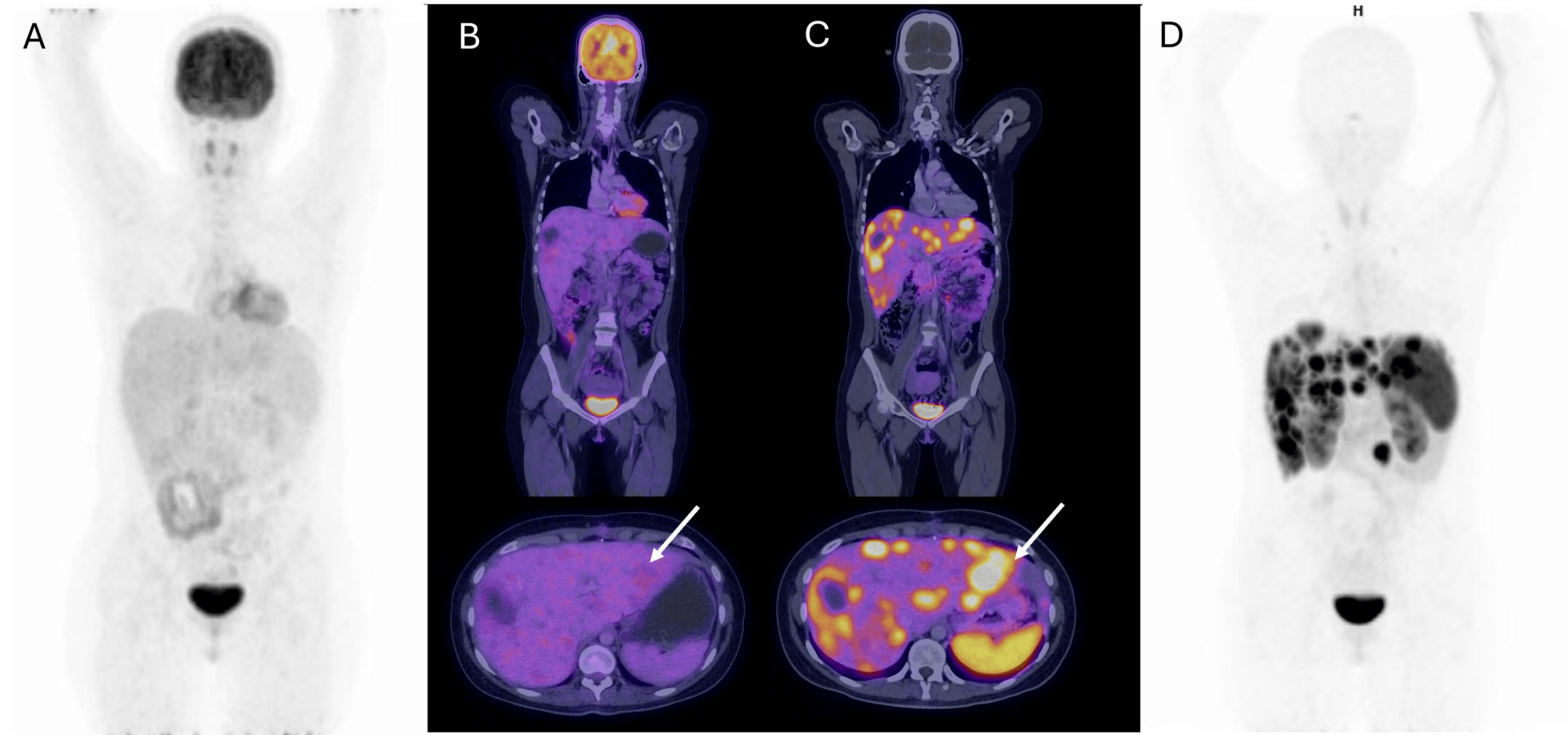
Dual positron emission tomography fused with computed tomography Dual positron emission tomography fused with computed tomography (PET/CT) A) PET/CT with (^18^F) Fluorodeoxyglucose (FDG) maximum intensity projection (MIP) with no sites of abnormal uptake of the radiotracer (B). ^18^F-FDG PET/CT axial and coronal fused images show multiple liver lesions without metabolic activity (white arrow). (C) (^18^F) AlF-NOTA-octreotide PET/CT axial and coronal fused images; multiple liver lesions are identified with overexpression of somatostatin receptors (white arrow). (D) (^18^F) AlF-NOTA-octreotide PET/CT MIP shows multiple positive hepatic lesions; a focal uptake of the radiotracer was also observed in small intestine (proximal ileum). The dual image findings correspond to well-differentiated neuroendocrine neoplasia.
